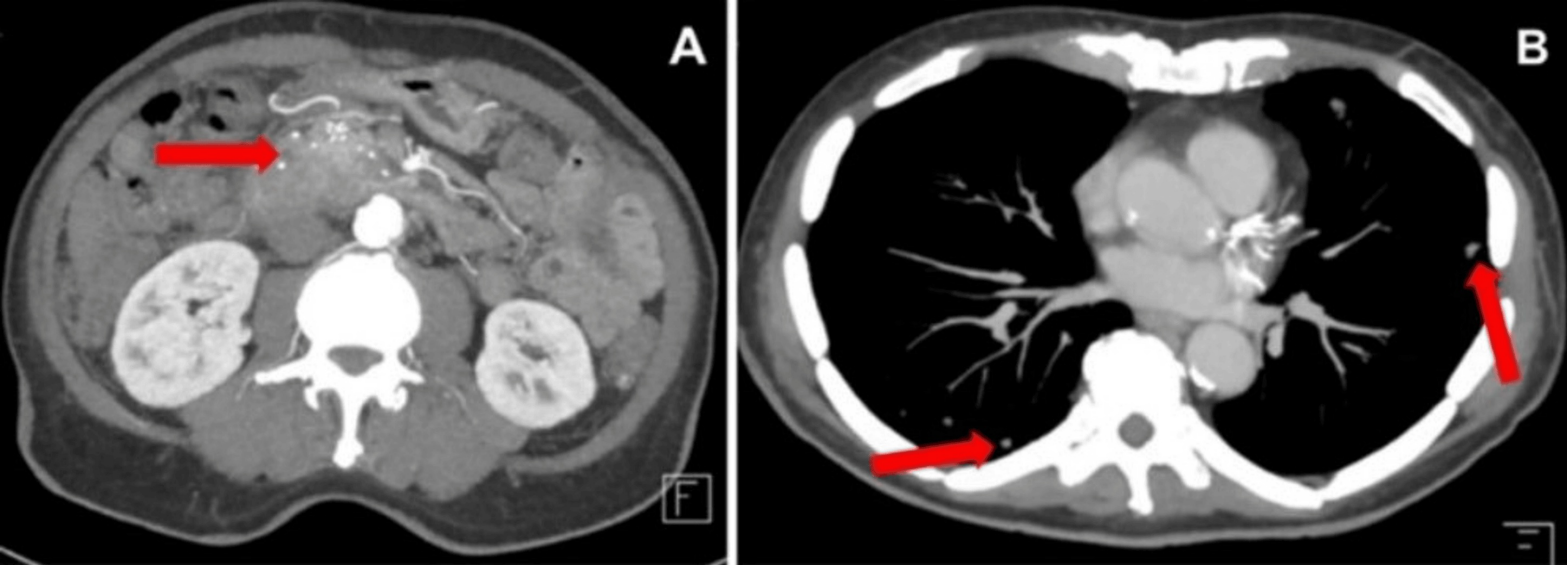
Patient’s initial CT imaging with contrast. (A) CT scan of the abdomen demonstrating marked biliary dilatation, an overdistended gallbladder, and a 3.8 × 2.1 cm mass in the head of the pancreas (arrow). (B) CT scan of the thorax demonstrating multiple pulmonary nodules concerning for possible metastasis (arrows).
